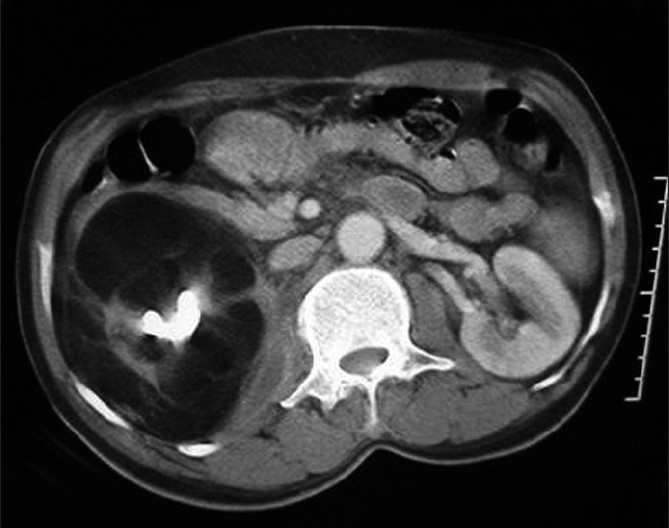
Contrast-enhanced CT of abdomen shows replacement of the right kidney by fat with a large central hyperdense calculus. A small posterior perinephric collection is noted extending into the adjacent psoas. Thin residual strands of renal tissue are noted
